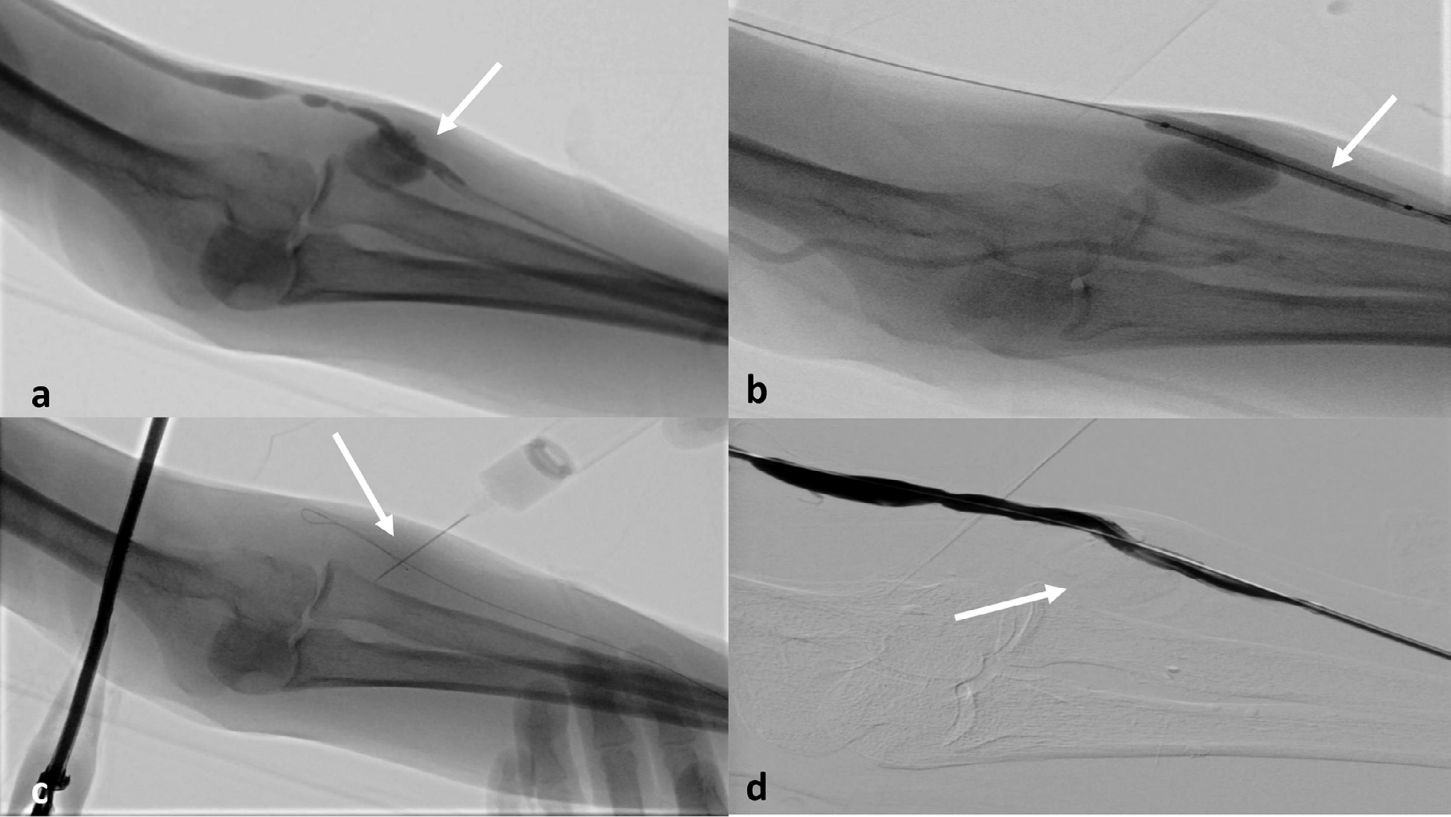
The diagnostic angiography confirmed the presence of pseudoaneurysm in the draining vein (a). A balloon was positioned and inflated across the pseudoaneurysm basis (b) and then, under ultrasound guidance, the pseudoaneurysm was punctured with a 21-gauge needle and 2 mL of thrombin was real-time injected, until to complete filling the pseudoaneurysm (c). At the final check, the pseudoaneurysm was completely excluded from the circulation (d).
